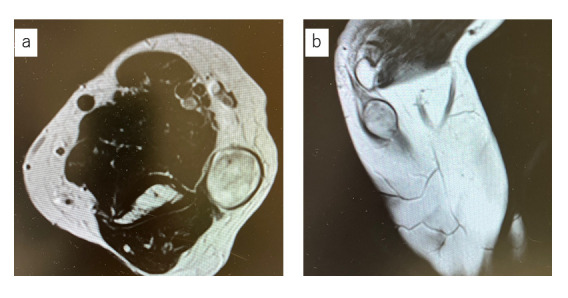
T2-weighted radiographs acquired by magnetic resonance imaging (MRI). (a) The target sign was observed in the axial view at the left elbow. (b) The target sign and split fat sign were observed in the sagittal view at the left elbow.
